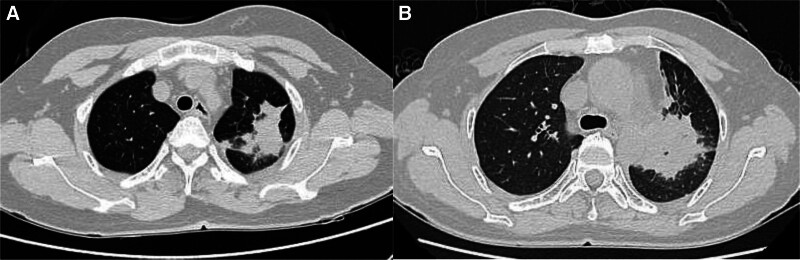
Above are the chest CT images, of which part (A) is the first chest CT image in our hospital, with a lesion measuring approximately 5.1 × 3.6 cm; Part (B) is the second chest CT image, with a lesion measuring approximately 5.9 × 4.6 cm. CT = computed tomography.
